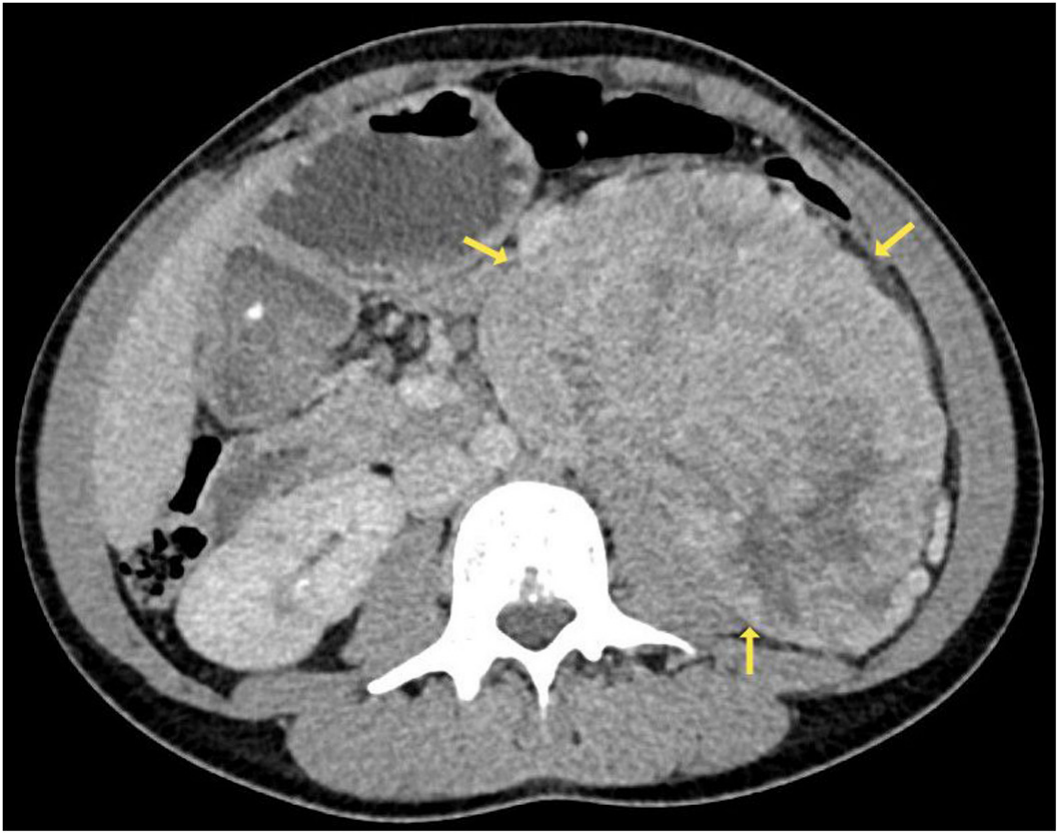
Enhanced CT demonstrating a 13.4 × 9.1 × 15.3 cm lobulated, heterogeneously enhancing retroperitoneal mass with associated left-sided hydronephrosis.
